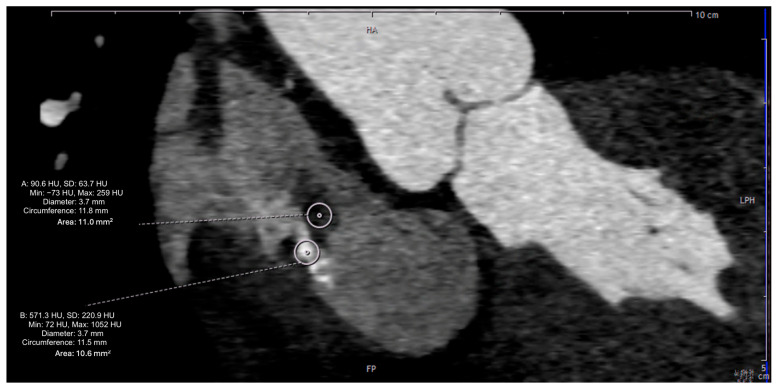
Cardiac CT image demonstrating tissue characterization using Hounsfield Units (HUs).
